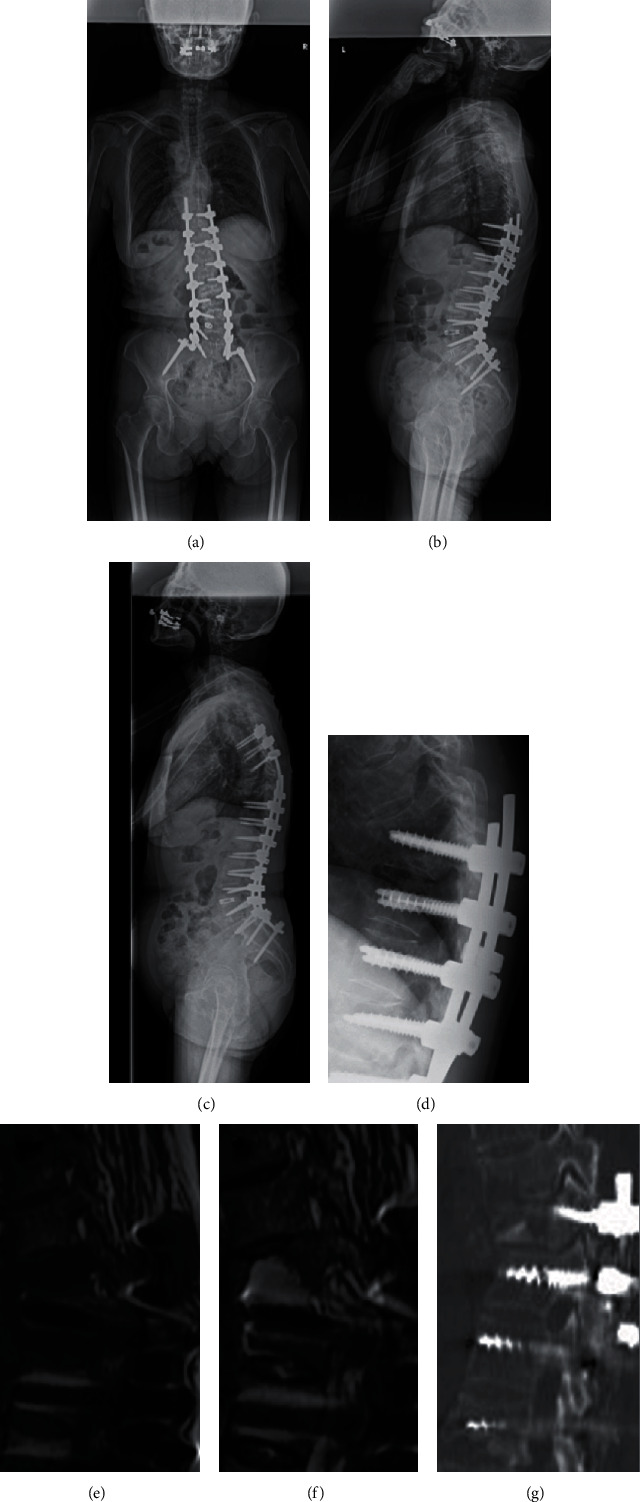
The 72-year-old female patient received PSF (Th10 to ilium). (a) Immediate postoperation whole spine posterior-anterior (PA) standing radiograph demonstrates normal coronal alignment. (b) Immediate postoperation whole spine lateral standing radiograph demonstrates normal sagittal alignment. (c) Immediate postrevision lateral view shows normal sagittal alignment. (d) PA view at readmission shows severe PJK (PJA 33.4 deg.). (e) T1-weighed MR image (T1WI) around the UIV area shows bone destruction of both UIV and UIV+1 vertebra. (f) T2WI around the UIV area shows fluid correction around the UIV vertebra. (g) CT scan around the UIV area shows bone destruction of both UIV and UIV+1 vertebra.
